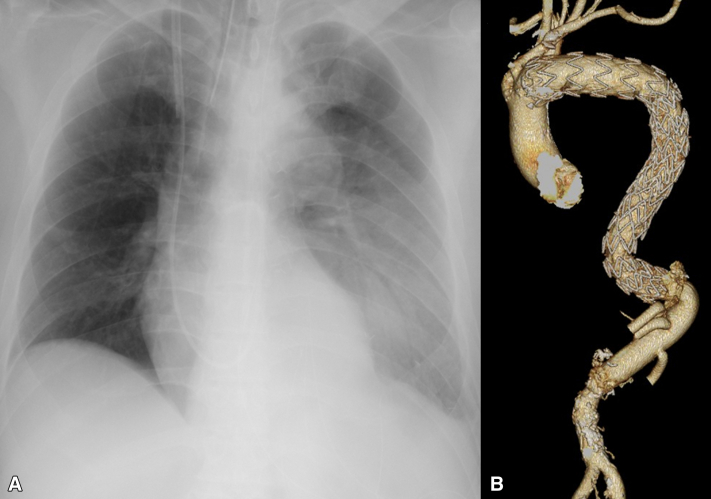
A, Immediate postoperative radiograph of the chest showing preserved lung expansion. B, Postoperative computed tomography scan confirming proximal anastomosis and graft continuity.
